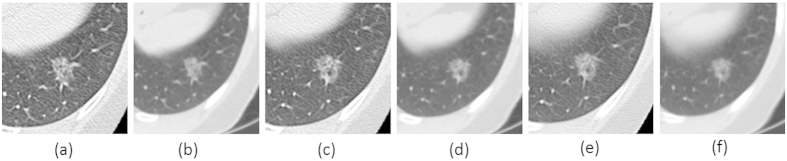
A lung tumor captured on one CT scan reconstructed at 6 different imaging settings: 1.25mm slice thickness with the lung reconstruction algorithm (sharp image) (1.25L) (a) and the standard reconstruction algorithm (smooth image) (1.25S) (b); 2.5mm slice thickness with lung reconstruction (2.5L) (c) and standard reconstruction (2.5S) (d); 5mm slice thickness with lung reconstruction (5L) (e) and standard reconstruction (5S) (f).
